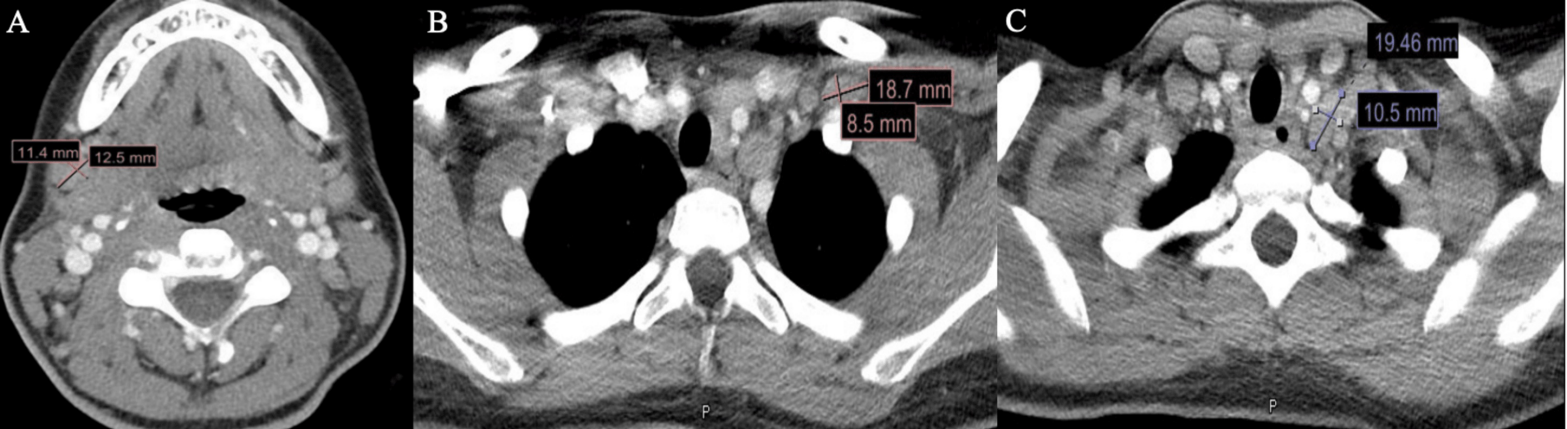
Computed Tomography (CT) of the Neck CT of the neck demonstrating cervical lymphadenopathy. A few prominent bilateral cervical and submandibular lymph nodes are visible, with the largest measuring 12.5 × 11.4 mm in the right submandibular level (labeled in A), 18.7 × 8.5 mm in the left supraclavicular level (labeled in B), and 19.46 × 10.5 mm in the left lower cervical level (labeled in C).
